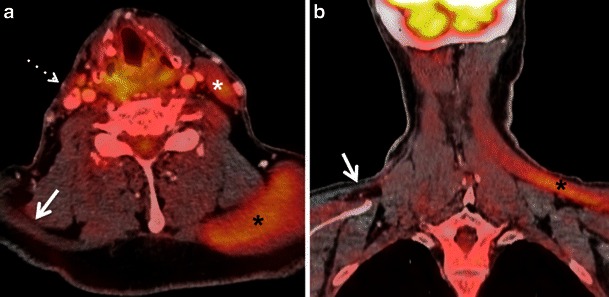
a Axial PET/CT image reveals compensatory FDG uptake of the left sternocleidomastoid muscle (*white asterisk*) and of the ipsilateral trapezius muscle (*black asterisk*) due to right spinal accessory nerve (XI) paralysis caused by a base skull adenocarcinoma. Also note atrophy of the right sternocleidomastoid muscle (*dotted arrow*) and of the right trapezius muscle (*arrow*). b Corresponding coronal PET/CT image depicts atrophy of the right trapezius muscle (*arrow*) and compensatory increased uptake of the left trapezius muscle (*black asterisk*)
